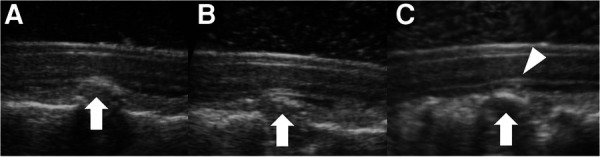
Intraoperative ultrasonography. After laminectomy. (A) The spinal cord is compressed anteriorly by ossification of the posterior longitudinal ligament (OPLL) (arrow). (B) Before resection of the PLL. After partial osteotomy of the posterior vertebra adjacent to the targeted OPLL and before PLL resection, the OPLL decreased (arrow). (C) After PLL resection. The OPLL further moves anteriorly (arrow), and a space between the spinal cord and dura mater is clearly detected (arrowhead).
